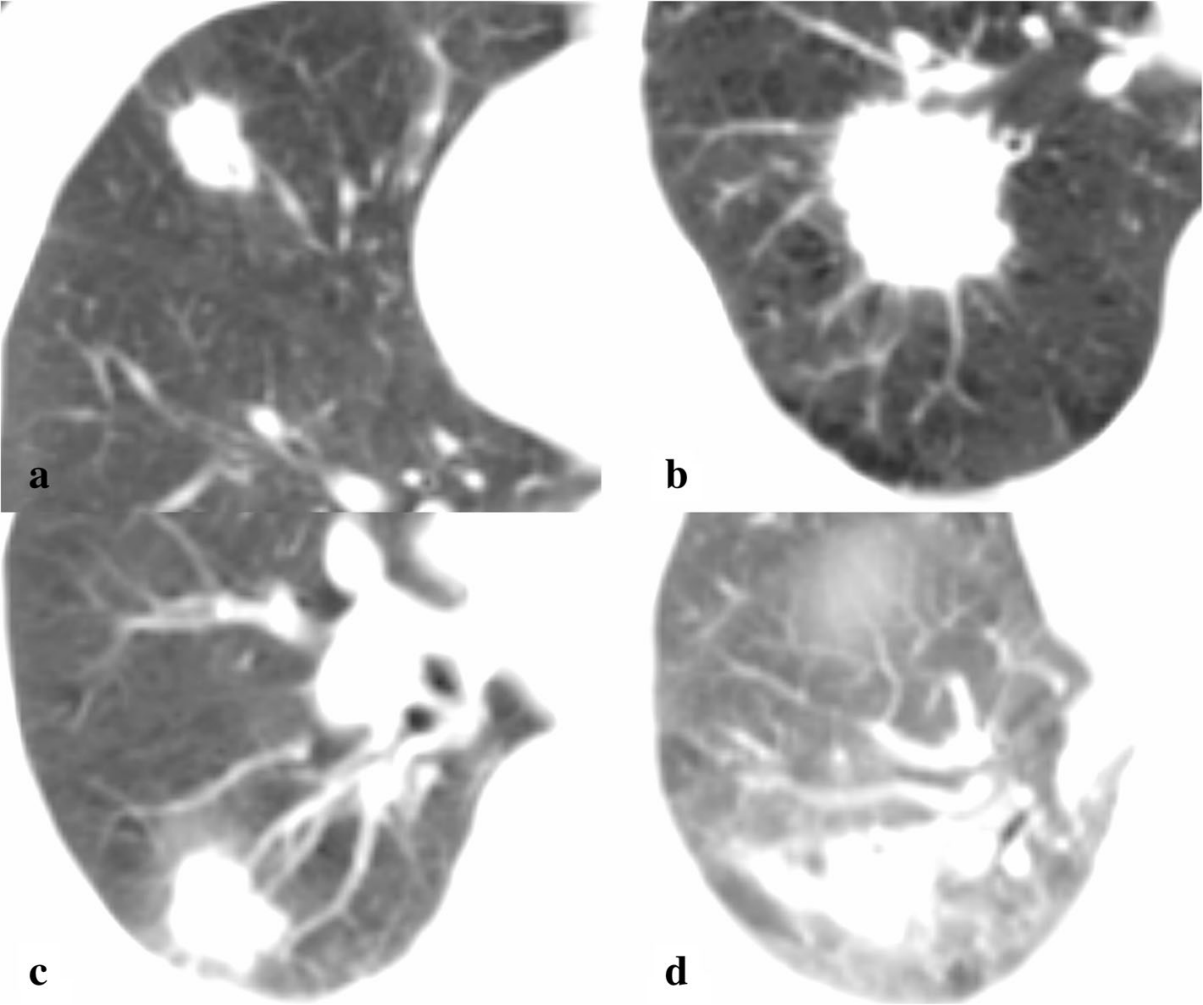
CT images of the four patients with typical tumor characteristics. a Patient with an off-CW small tumor; b Patient with an off-CW large tumor; c Patient’s tumor was adjacent to the CW; and d Patient at a high risk of RIP
